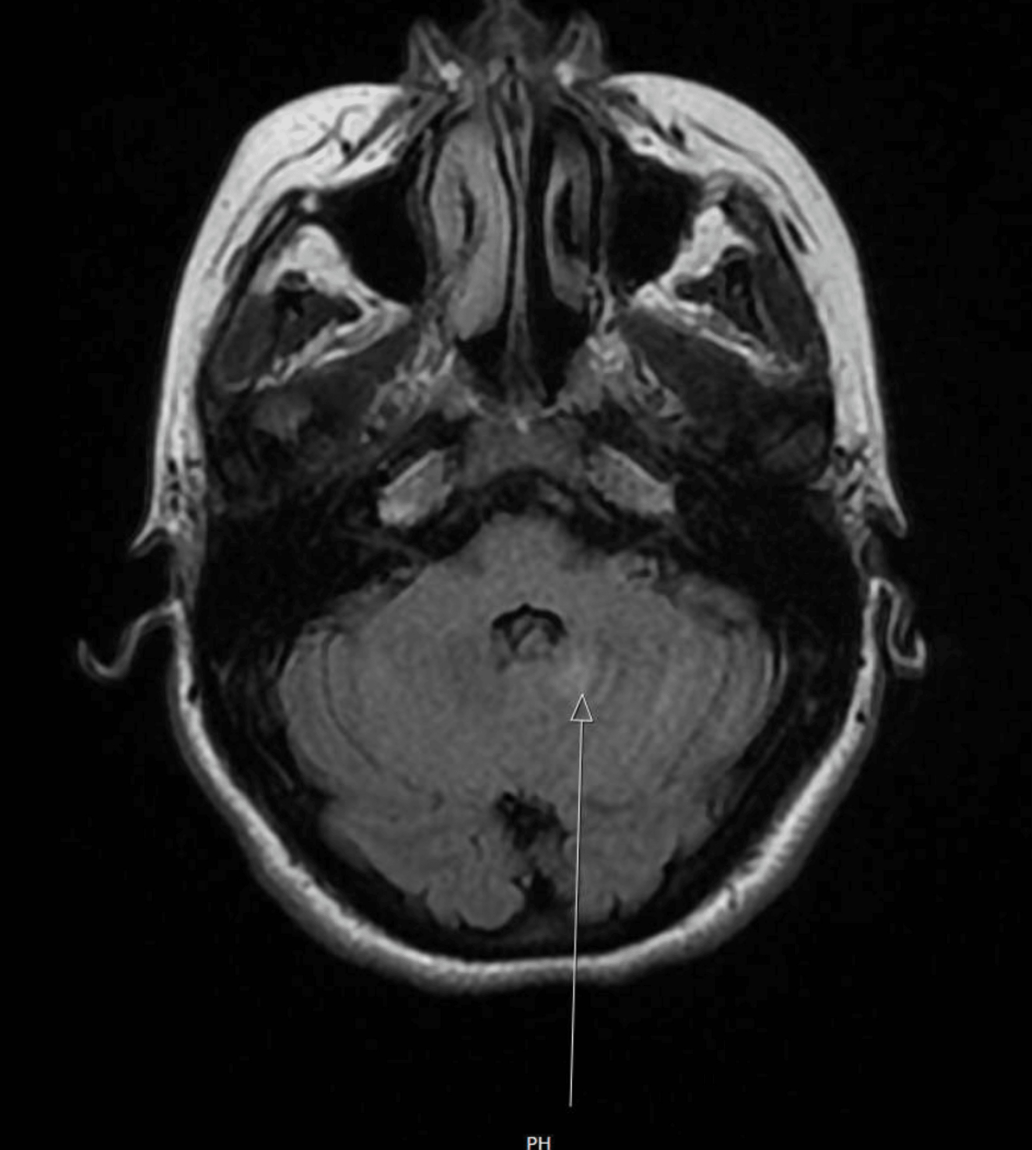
MRI brain demonstrating 1 cm focus of T2 hyperintense signal abnormality within the left cerebellar hemisphere, near the dentate nucleus (arrow), which increases suspicion for a possible demyelinating disease.
